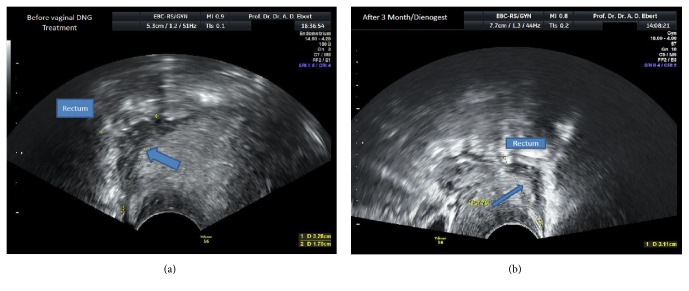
(a) Vaginal ultrasound appearance of the rectovaginal bowel involvement before the start of treatment in the symptomatic patient. (b) No change in the vaginal ultrasound findings after 3 months of vaginal dienogest administration (2 mg/d) in the patient who was now de facto asymptomatic.
